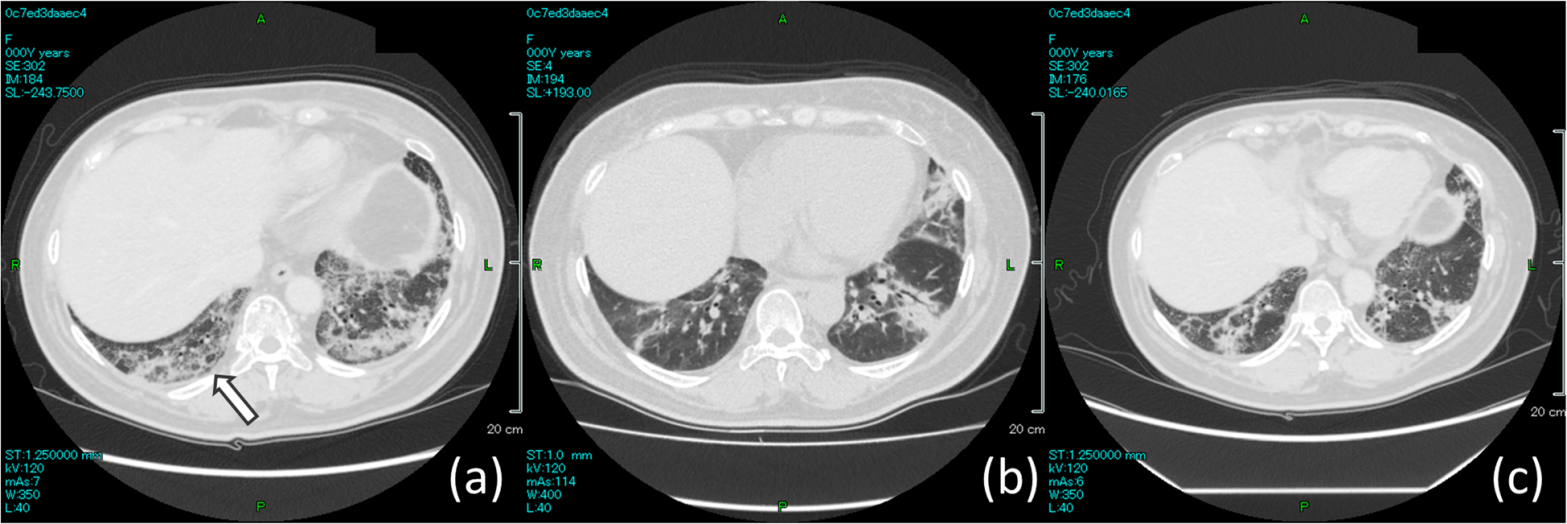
Computed tomography (CT) images of the lung field of Case 4. CT revealed the new appearance of ground-glass opacity, infiltrative shadows, and a rounded morphology (arrow) in the bilateral middle and lower lung fields (a). Fibrosis developed within 2 weeks (b) and then tended to disappear (c). The findings were highly suspicious of coronavirus disease 2019. Figure 3a was reprinted from reference [3]
